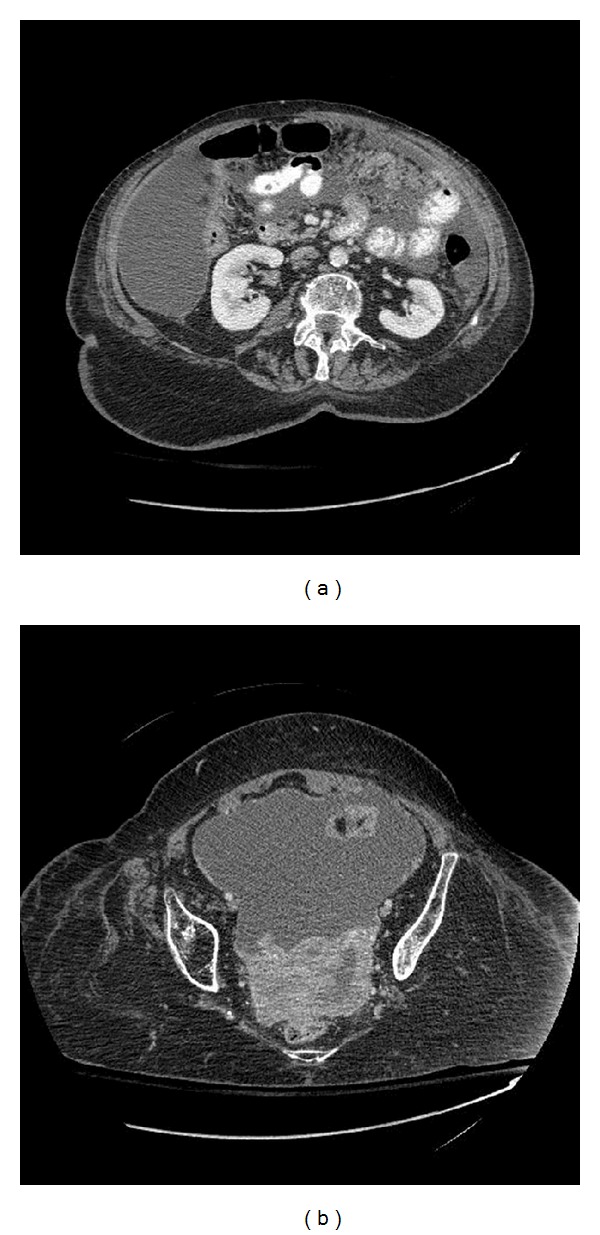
CT scan of the abdomen and pelvis shows ascites, nodularity in the peritoneal cavity (a), and prominent adnexal tissues (b).
